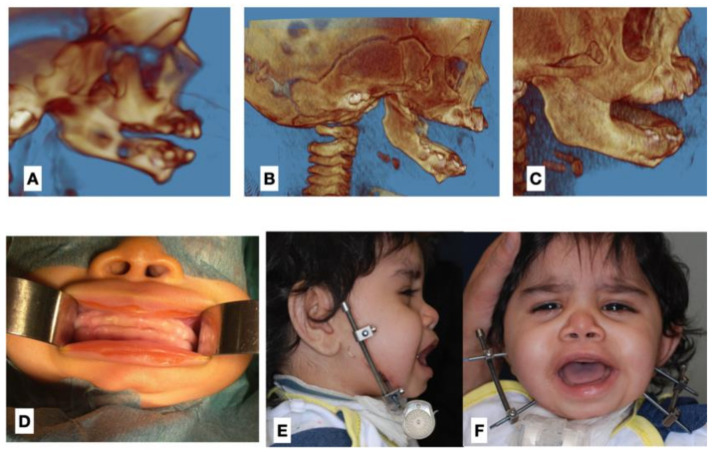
Representative photographs of a 2-year-old patient with bilateral TMJA. (A) 3D CT reconstruction of the zygomatico-coronoid ankylotic block diagnosed in infancy, (B) 3D CT scan reconstruction after early RAS performed at the neonatal age, (C) 3D CT scan reconstruction with visible recurrence at the age of 5 months, (D) intraoral photograph before treatment showing significantly decreased MIO, (E) lateral view during mandible osseodistraction performed as stage 3 surgery (after second RAS), (F) frontal view during osseodistraction showing improvement of the MIO.
